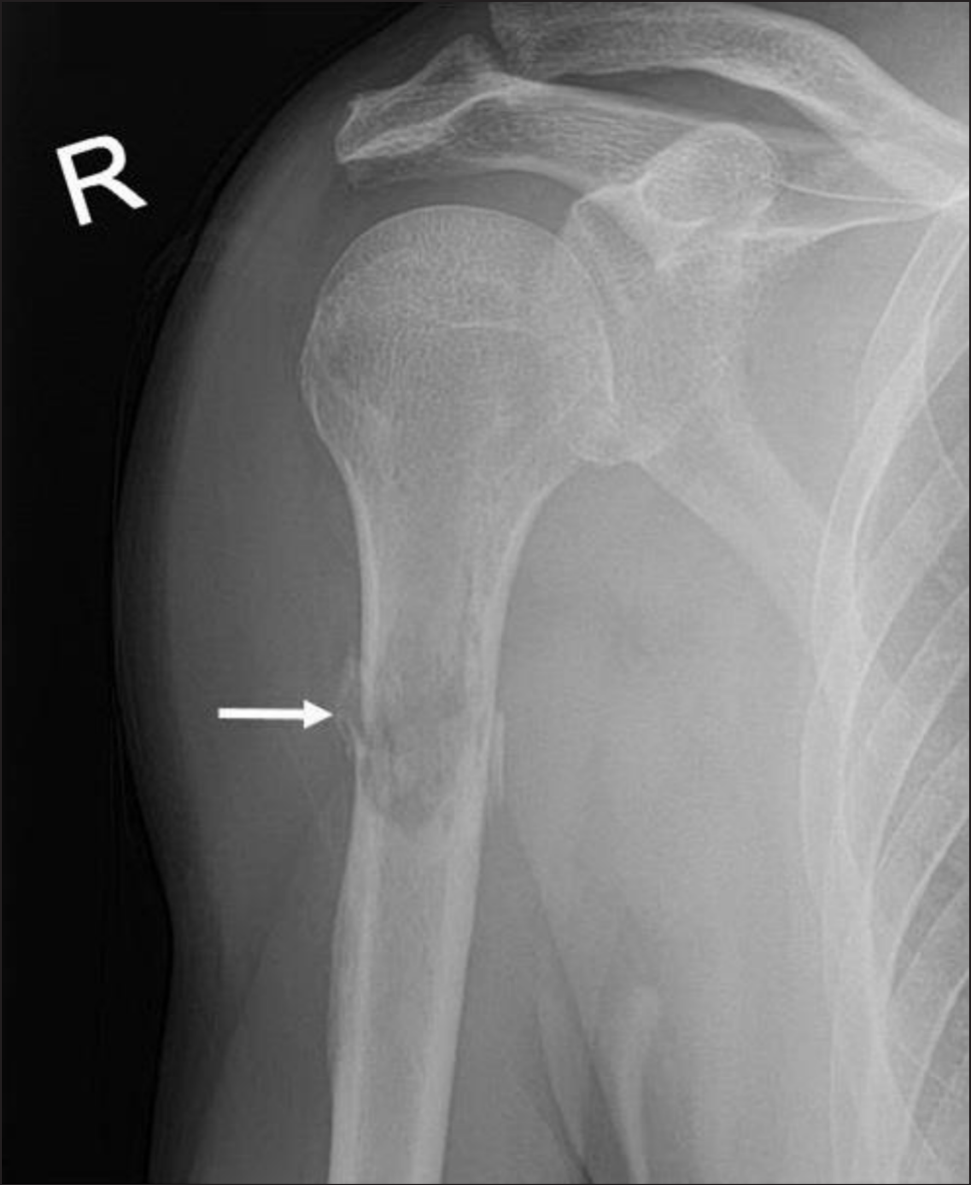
X-ray of the humerus: Fracture of the proximal humeral diaphysis (arrow) with a hazy appearance and a wide transition zone.
